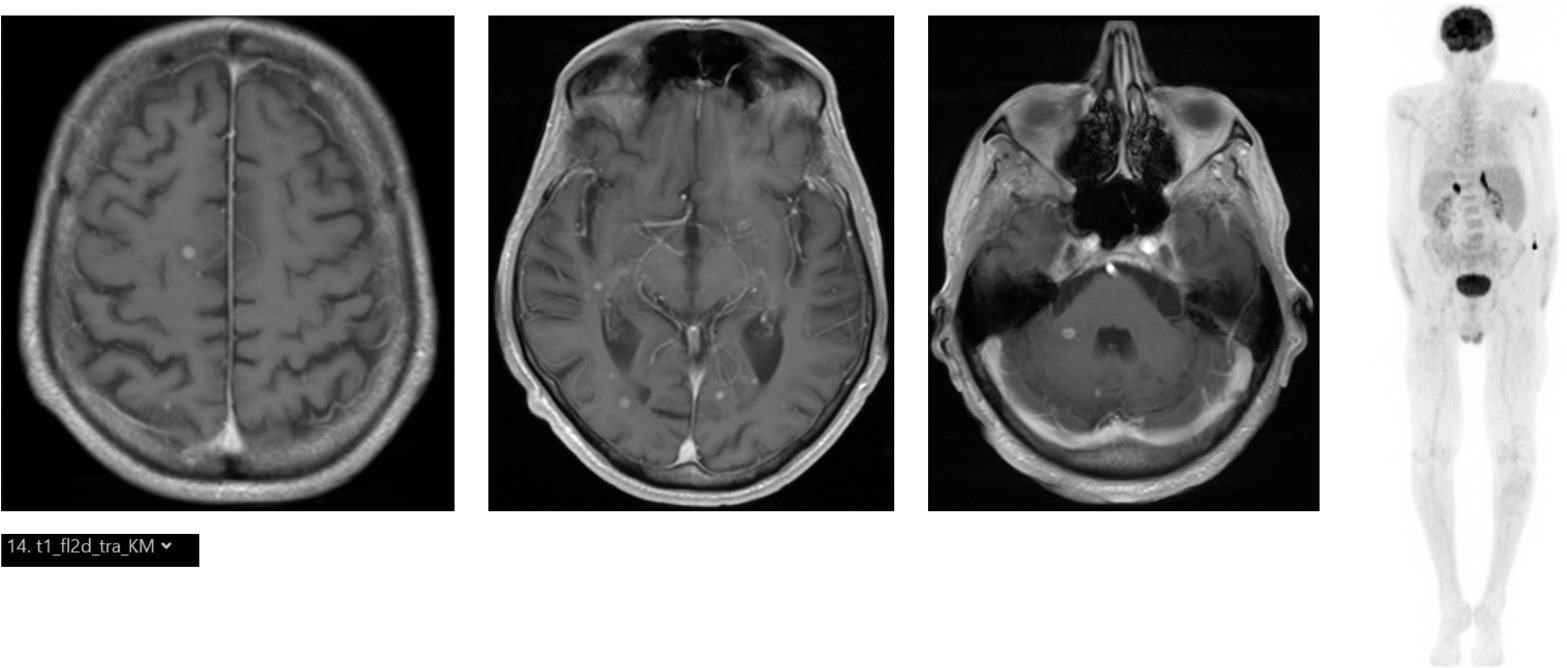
(Left) Cranial MRI with multiple contrast-enhancing lesions (T1). (Right) [^18^F]FDG PET/CT showing bilateral hypermetabolism of the adrenal glands.
